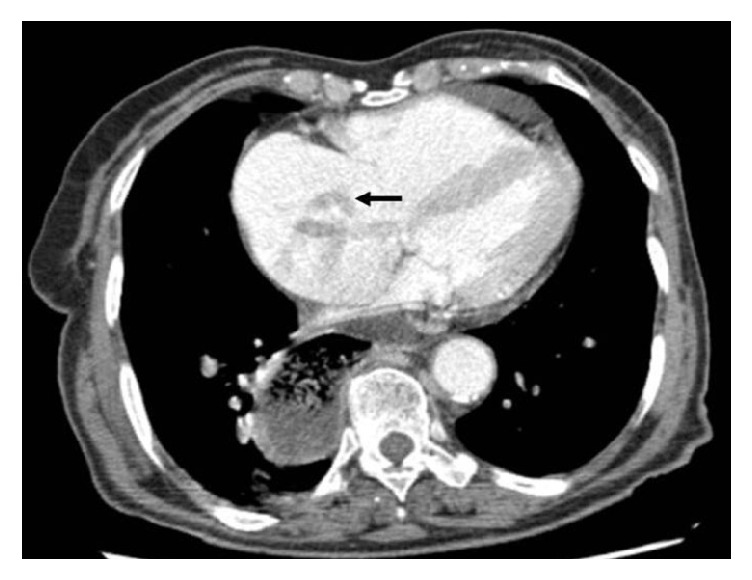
Computed tomography angiography revealing right heart chamber dilatation and the presence of a curled worm-like thrombus (type A) in the right atrium.
